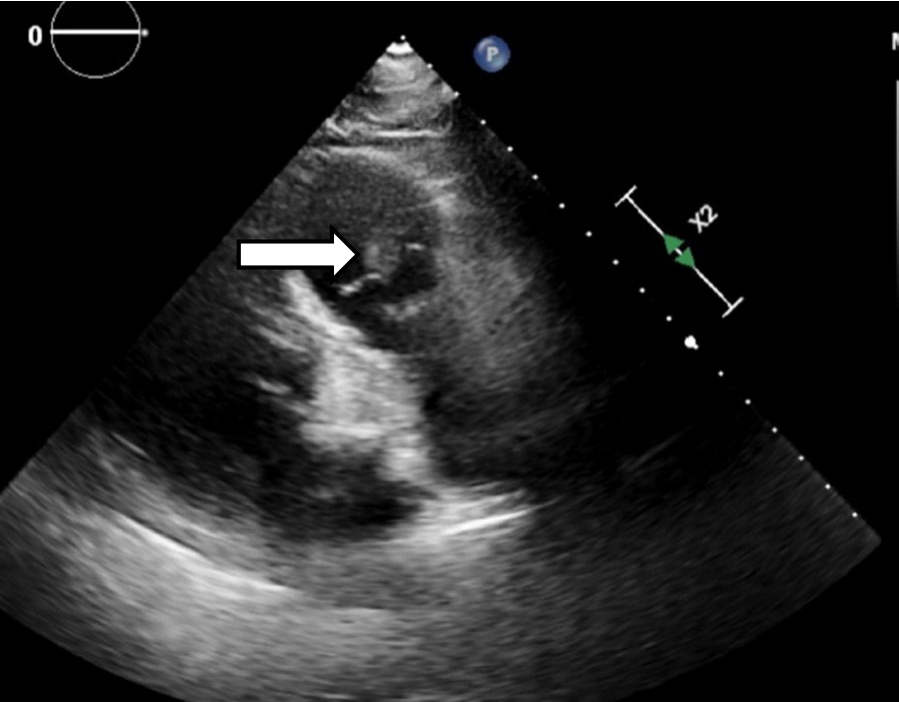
Cardiac ultrasound photo showing the pulmonic valve with vegetation (indicated by arrow)overlying the valve cusps
